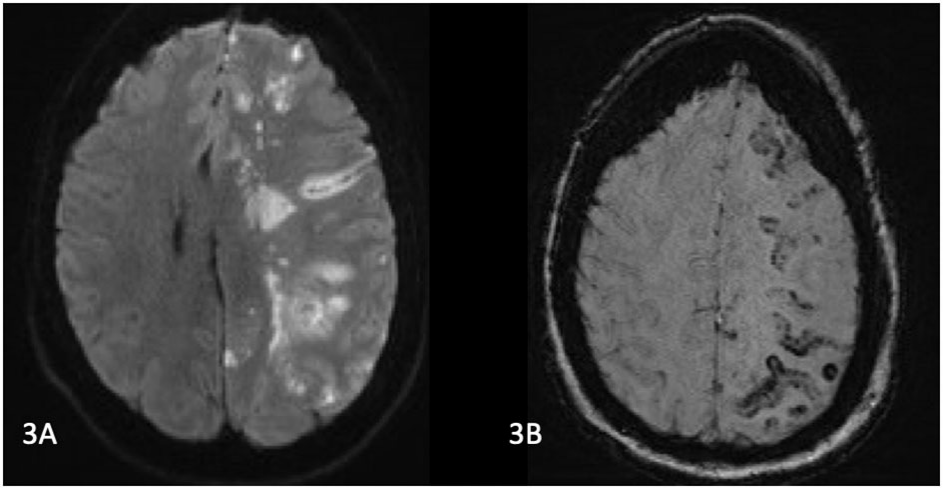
(A) MRI Brain on day 1 post operatively. Diffusion weighted images show left MCA territory infarction with a similar distribution of abnormalities on suspectibility weighted imaging) (B). MCA, middle cerebral artery; DWI, diffusion weight imaging; ECR, endovascular clot retrieval.
